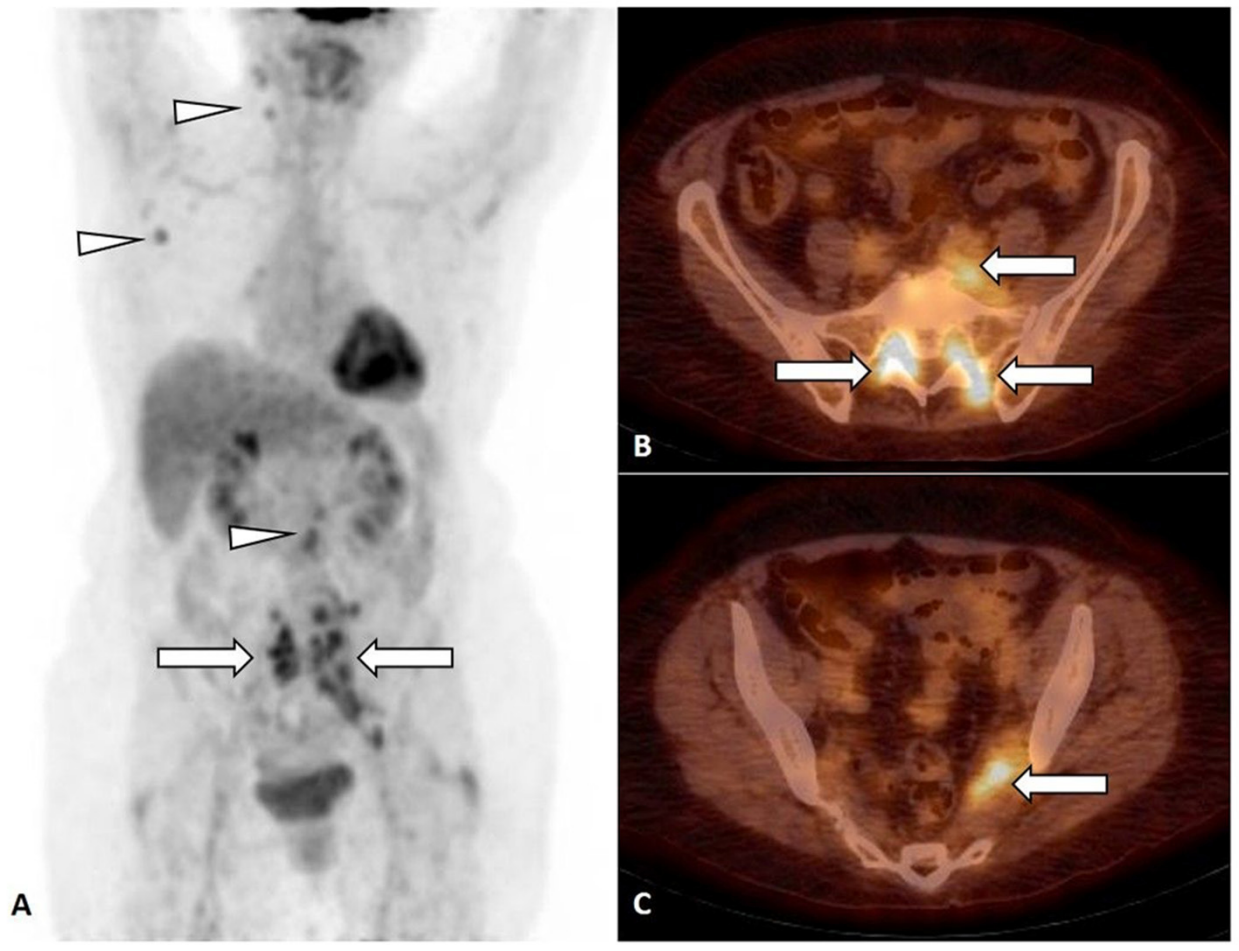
FDG PET, maximum intensity projection (MIP) (A) and FDG PET/CT, axial slice (B,C). Focal FDG uptake in lumbosacral plexus (LSP), predominantly on the left side [(A–C), arrow], in cervical, axillary, and retroperitoneal lymph nodes [(A), arrowhead].
